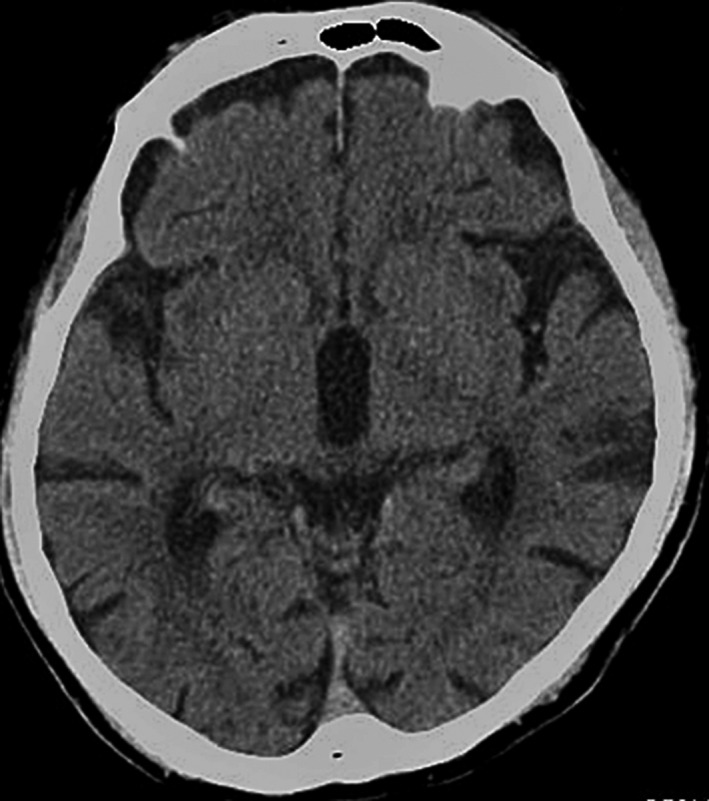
Isodensity to mild hyperdensity in the left putamen in head CT scan
